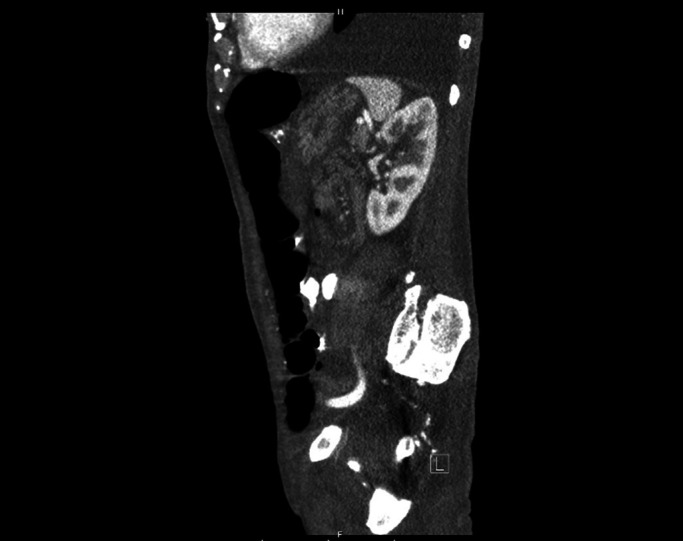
Abdominal computed tomography (sagittal projection) showing dilated loops of bowel and posterior displacement of a decompressed/retracted stomach.
